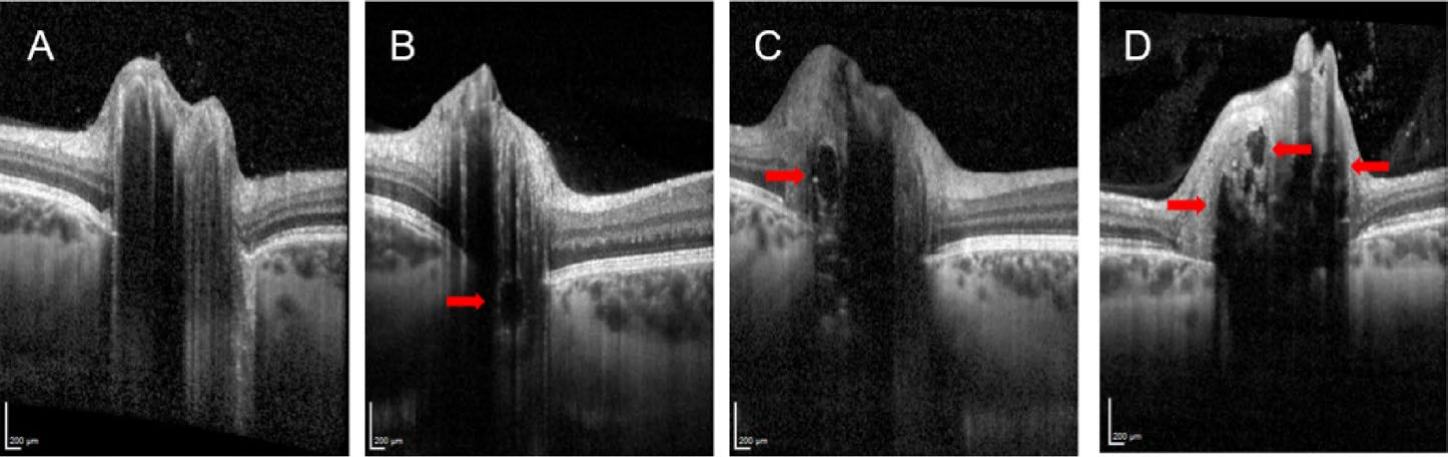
Representative samples for the OCT-based the classification system for ODD as described in the materials and methods section. Group A: deeply located drusen, detectable by ultrasound, exclusively (A). Group B: ODD discernible by OCT but still located below the level of Bruch´s membrane (B). Group C: single drusen (1–5) above the level of the Bruch´s membrane, regardless of whether deep drusen are also present (C). Group D: more than five ODD discernable by OCT above the level of Bruch membrane (D). The red arrow depicts ODD.
